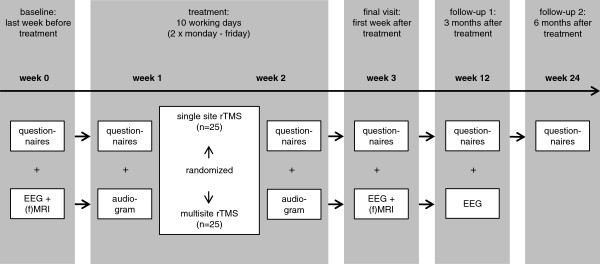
Visit schedule. EEG, electroencephalography; (f)MRI, (functional) magnetic resonance imaging; rTMS, repetitive transcranial magnetic stimulation.
